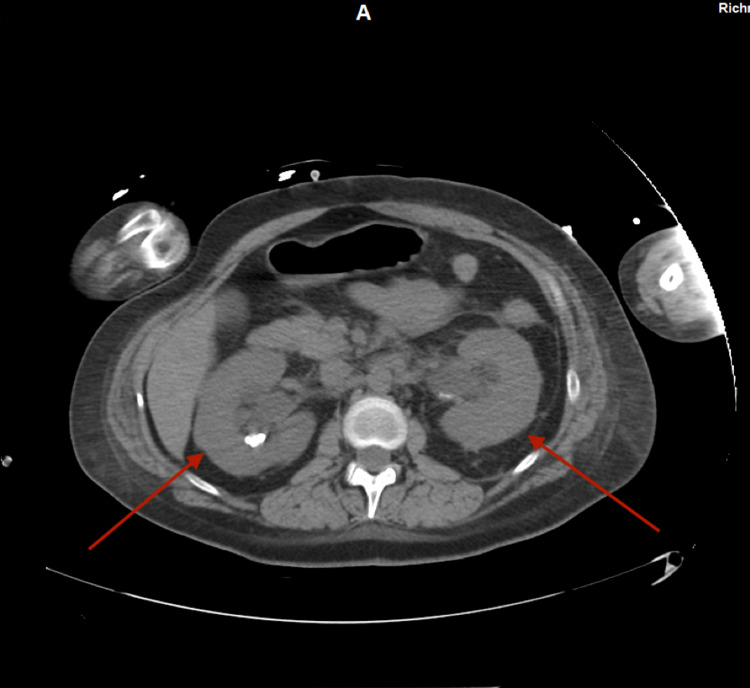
CT scan of the abdomen and pelvis showing bilateral hydronephrosis. CT: computed tomography
